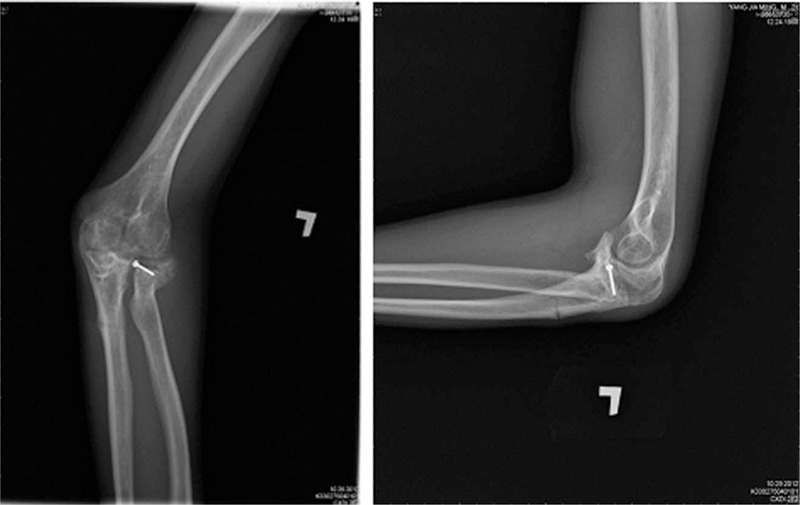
Five months after the removal of the internal fixation and after “breaking” sound, subsequent X-ray revealed a greenstick fracture of the left ulnar shaft.
